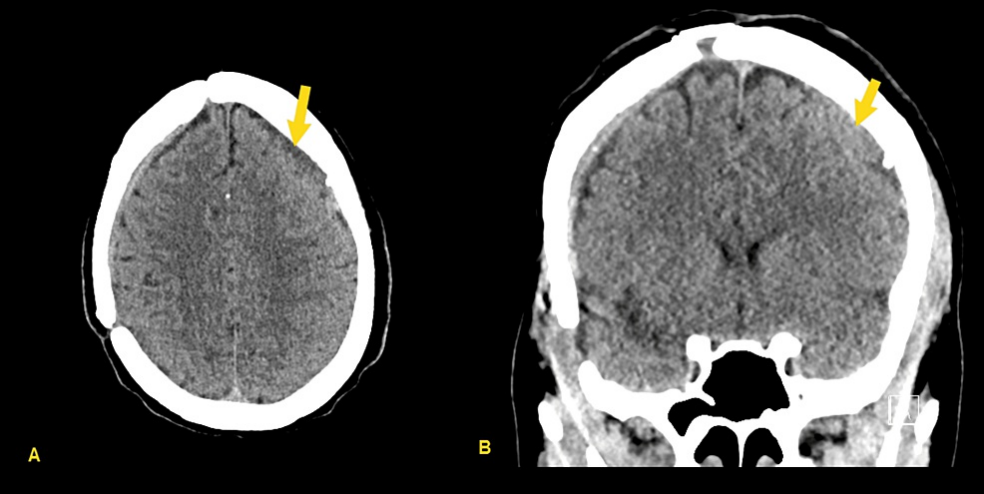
CT head 12 weeks after cranioplasty, showing the development of a new small acute right frontal subdural hematoma (SDH) (yellow arrows), suspected again to be spontaneous (A) Axial image, (B) Coronal image
